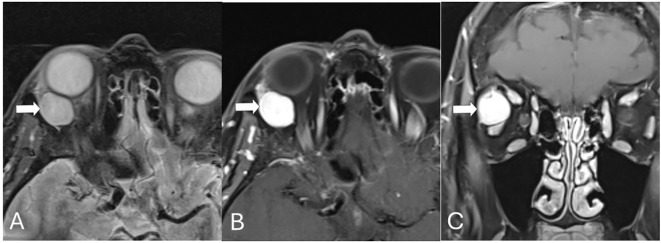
A 70-year-old woman with a right lacrimal adenoid cystic carcinoma. (A) Axial T2 MRI shows a homogeneously hyperintense appearance of the right lacrimal mass (arrow). (B, C) Axial and coronal T1 post-contrast MRI shows an oval-shaped mass with homogeneous enhancement (arrows).
